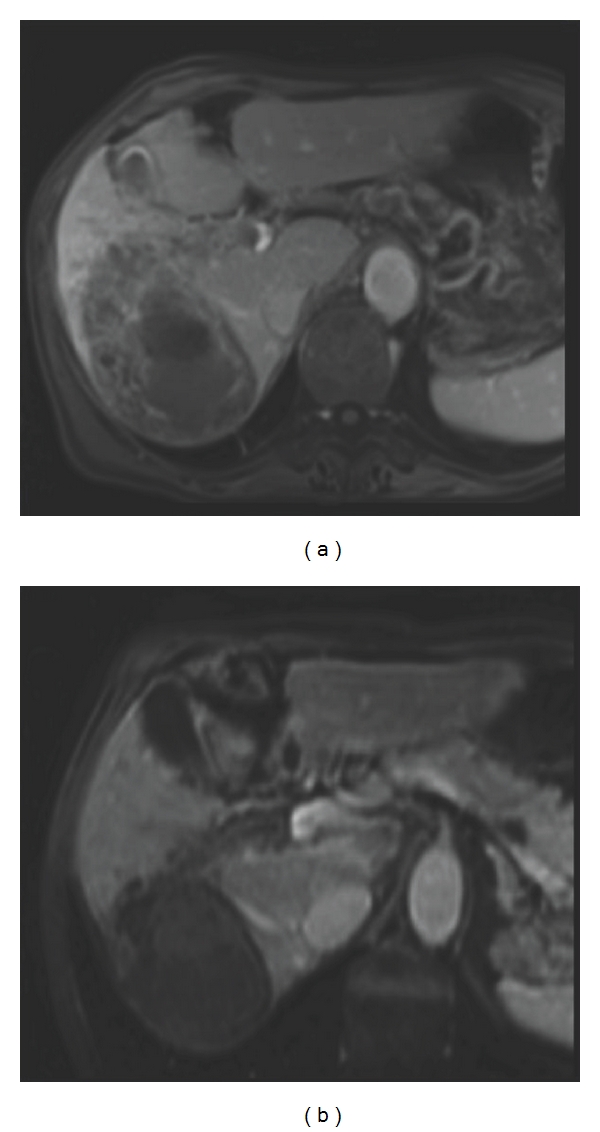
MRI of patient 1.* (a) Before treatment. (b) After 9 months of treatment with sorafenib.
